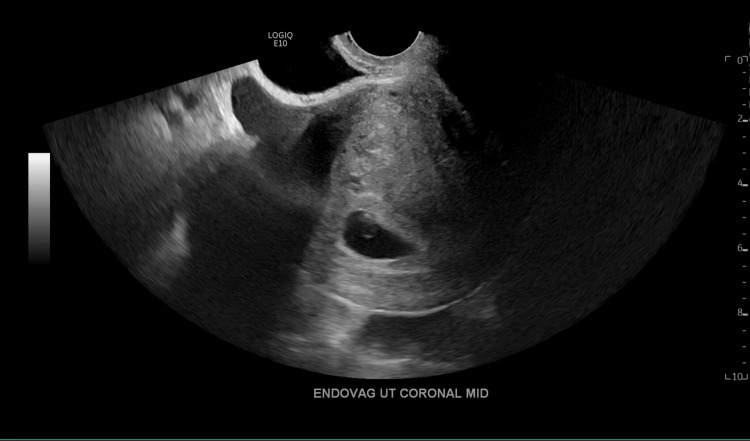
Transvaginal ultrasound in the mid-coronal plane demonstrating evidence of an intrauterine pregnancy with a visible gestational sac and yolk sac.
